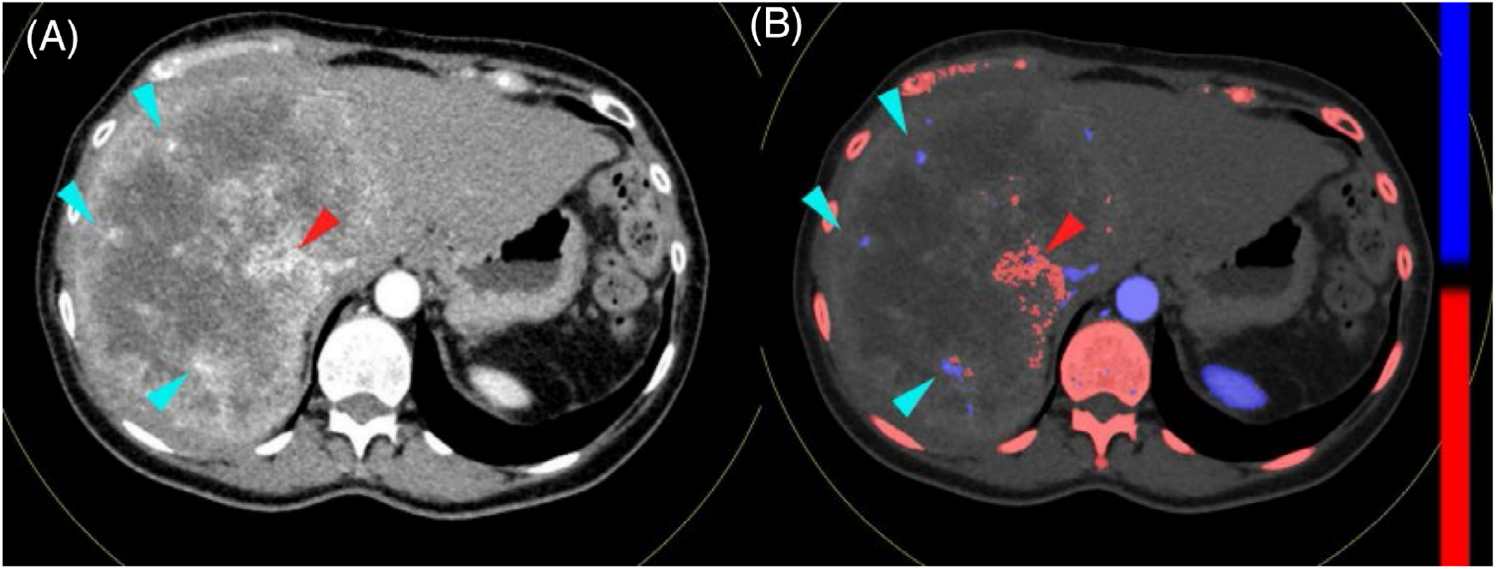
CT and dual‐energy CT. Female, metastasis from colorectal cancer. A Mixed image. The enhancing arteries within the lesion (light blue arrowheads) have density similar to intralesional calcifications (red arrowhead). B Material labeling. The material decomposition evaluates the different attenuation curves of the basis materials, allowing for material labeling (iodine: blue map and arrowheads; calcium: red map and arrowheads).
